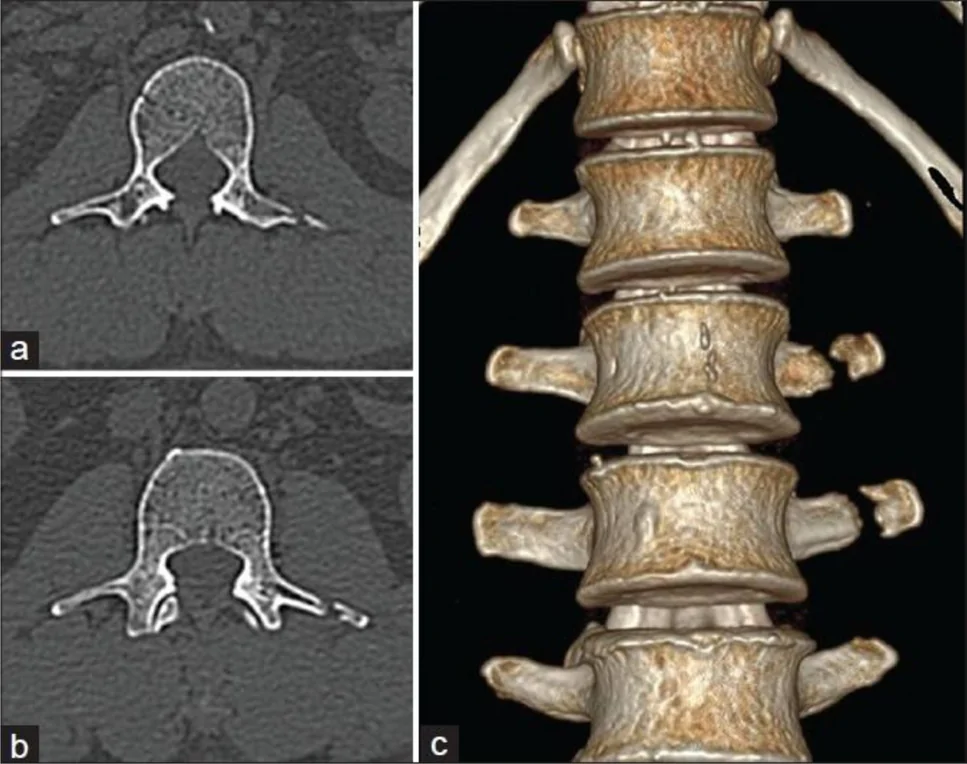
(a) and (b) Helical CT scans with (c) 3D reconstruction showing the fracture segments [23]; reproduced from Hegde et al. [23], with permission
